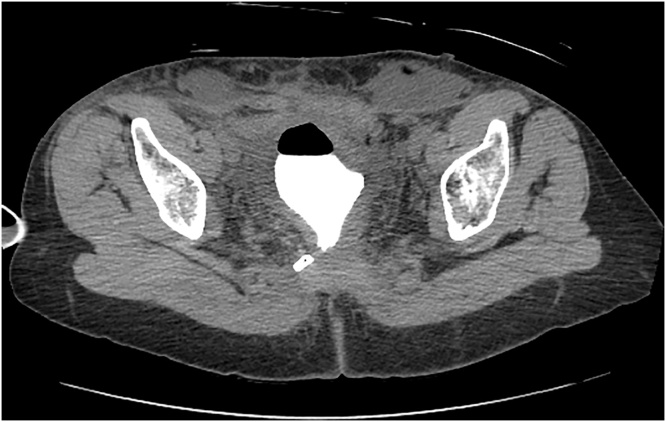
Cystogram (07/01/2020). A CT cystogram shows no contrast extravasation from the bladder. The closed suction drain remains in place. Lymphocoeles are present in the inguinal sites.
